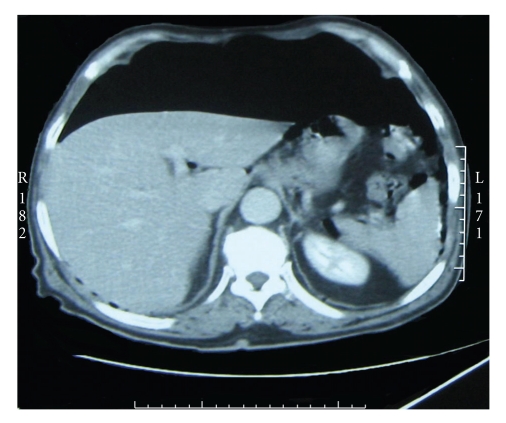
Abdomen CT with free intra-abdominal air.
